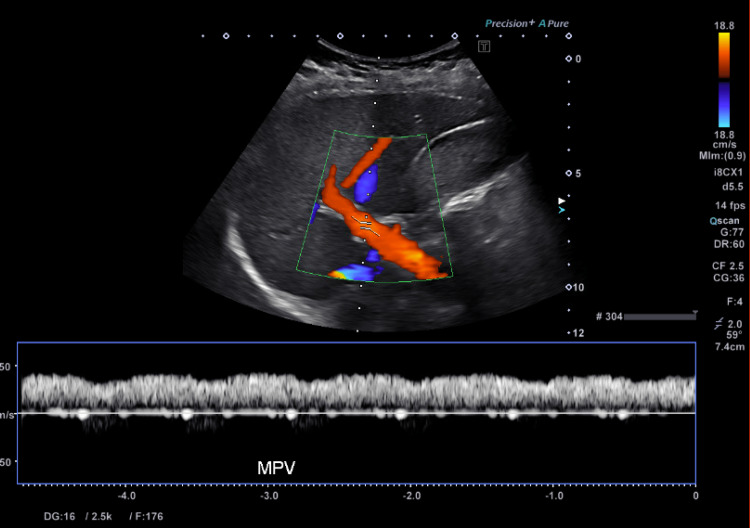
Normal abdominal ultrasound with Doppler. The image shows normal blood flow through the main portal vein. MPV: main portal vein
